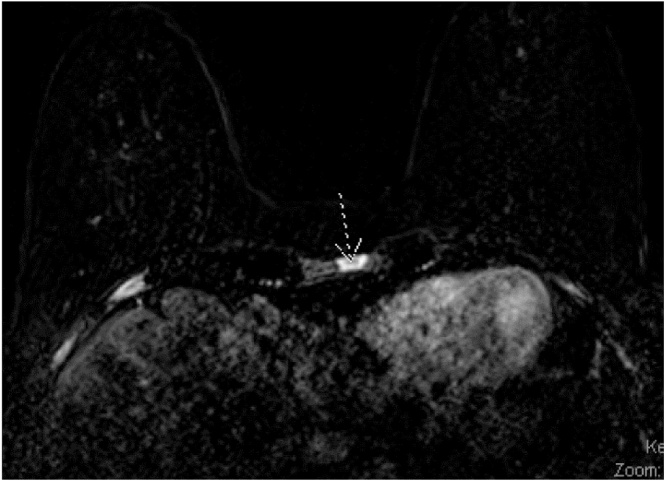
Sternal osseous metastasis suspicious masses on breast MRI.
